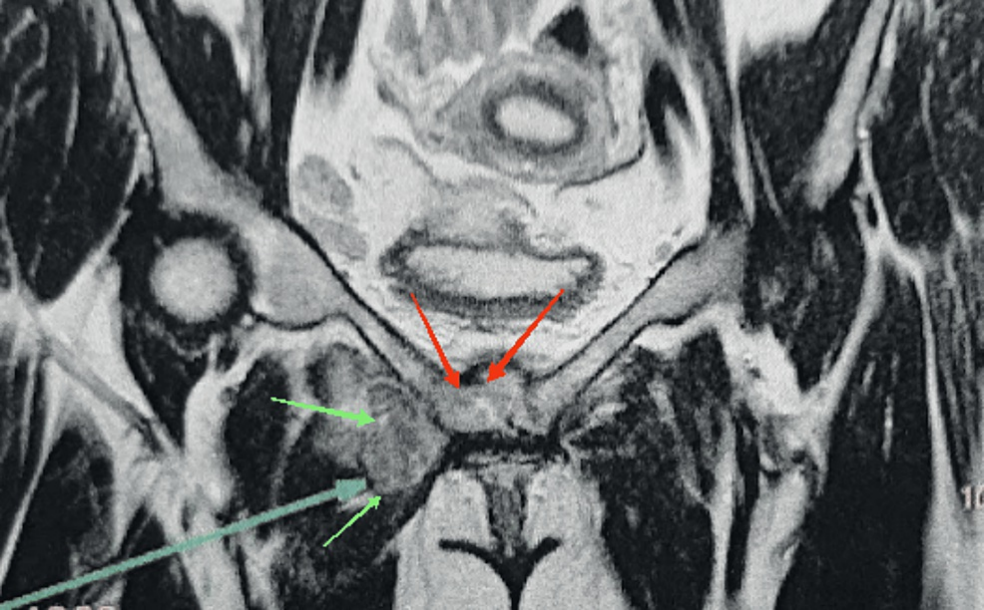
Pelvic MRI showing lysis of the horizontal branch of the pubis (red arrow) and a cystic image opposite (green arrows)
